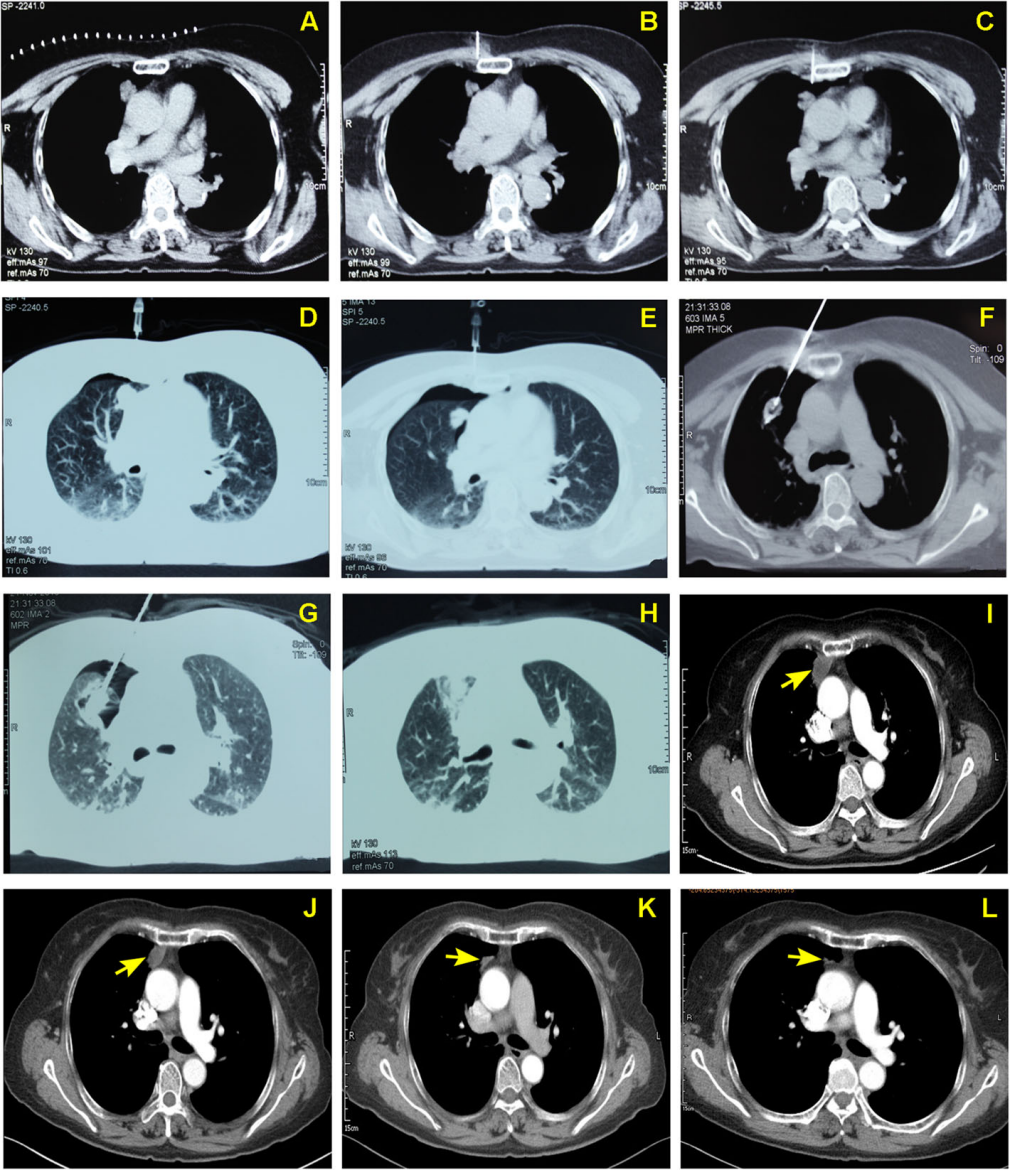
Artificial pneumothorax adjuvant RFA of pulmonary metastases contiguous to the mediastinum (a 67-year-old man with a metastatic lesion in the superior lobe forepart of right lung). Tumor size, 1.5 × 1.2 cm. a Before ablation, chest CT imaging was performed to evaluate the anatomic relationship between tumor and peripheral cardiovascular structures. b Subsequently, a 22-G needle tip was used to create a puncture that reached the outer edge of pleura for injection of 1–2 ml saline. c The needle tip entered into the pleura, and the saline in the tube flowed into the cavity. d-e CO_2_ gas was administered gradually with a syringe until the tumor was separated from the mediastinum. f CT image during RFA showed the electrode inserted into the tumor and located away from the mediastinum by proxy of artificial pneumothorax. g The ablation zone gradually increased following the RFA procedure. h After RFA, the pulmonary texture around tumor showed a circular exudation shadow with ground-glass appearance on CT image. i Contrast enhanced CT image 1 month after RFA showed no enhancement of the ablated tumor contiguous to the mediastinum. j-l The size of ablated tumor decreased gradually after RFA during follow up at 3, 6, and 12 months, respectively
